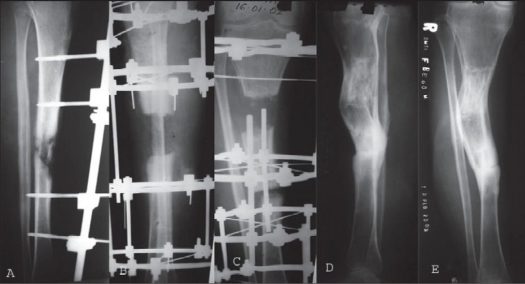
Conventional Ilizarov assembly. A) Open tibial fracture treated initially with debridement and unilateral external fixator. B) Second debridement and conventional Ilizarov frame application. C) Follow up X-ray depicting internal bone transport. D and E) X-ray post frame removal and fracture consolidation
